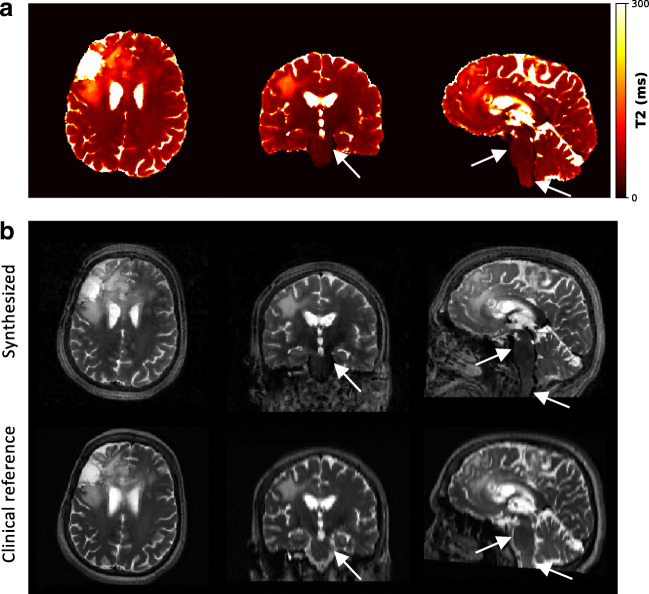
Sensitivity to physiological motion. Pulsating blood flow and thereby induced pulsation of the cerebrospinal fluid (CSF) can impact the T2 estimation (a) and subsequent synthesis of T2-weighted image contrasts (b) as observed in large vessels and in regions with high CSF pulsation, e.g., along the brainstem (white arrows)
